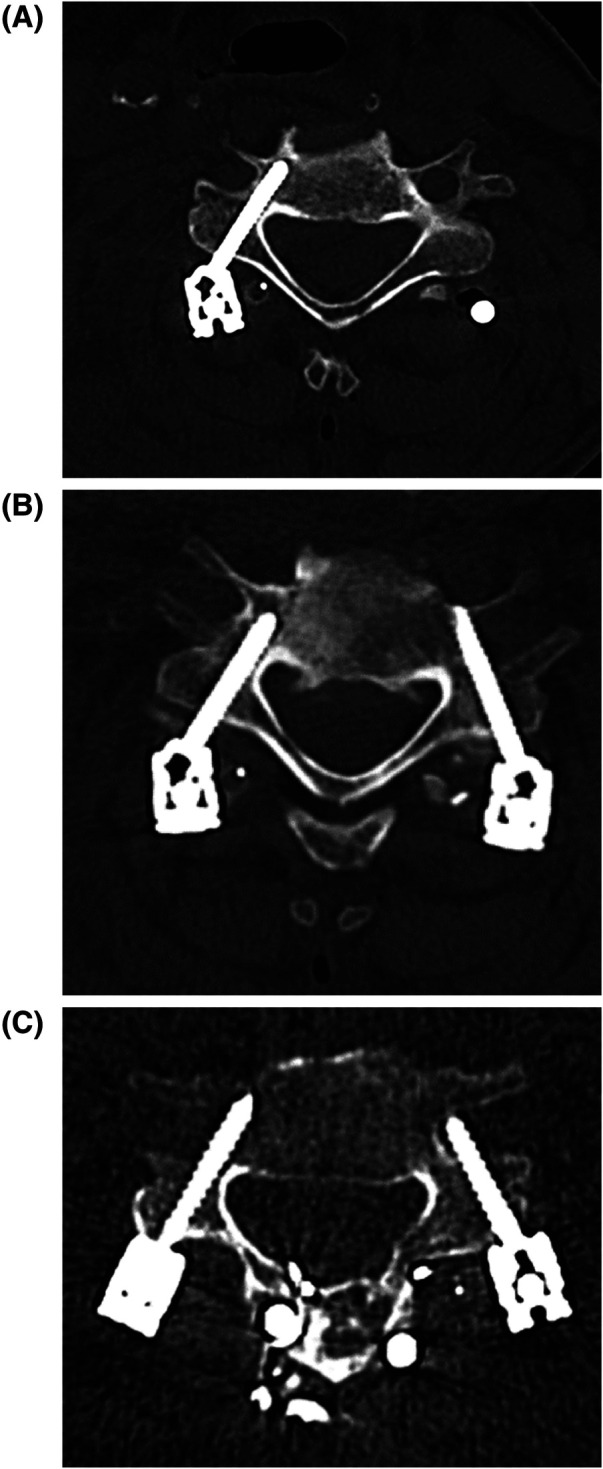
Accuracy of navigated PS placement on postoperative cervical CT. (A) Right side: PS level C5; accuracy Grade 1. (B) Left side: PS level C6; accuracy Grade 2; right side: PS level C6; accuracy Grade 1. (C) Left side; PS level C5; accuracy grade 3; right side: PS level C5; accuracy Grade 2.
